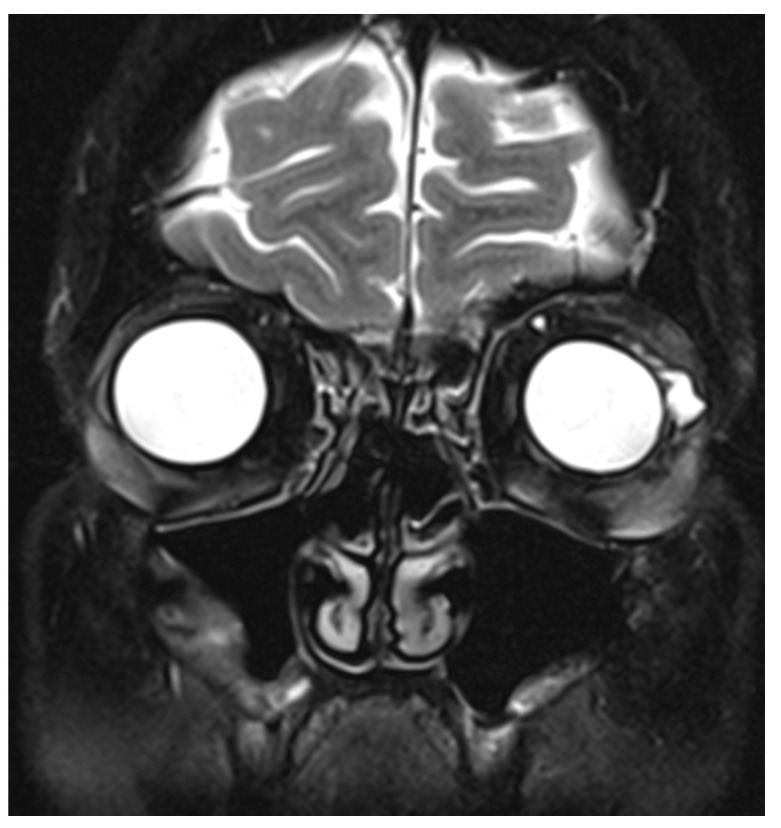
Coronal section of T2-weighted sequence of magnetic resonance imaging (MRI) of the orbits showing a cystic elevation above the left lateral rectus, likely to be a remnant of exposed hydrogel explant.
